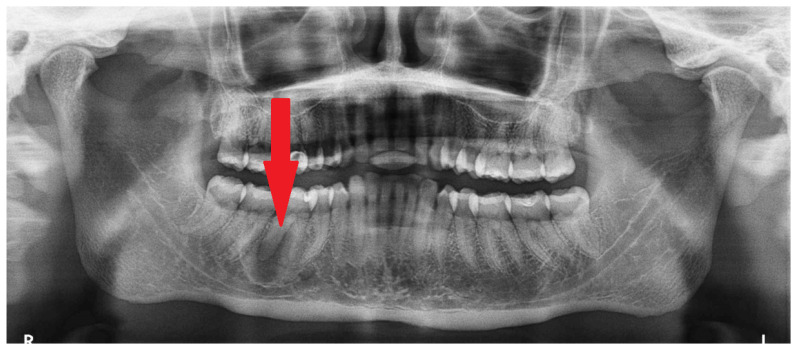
The occurrence of different irregular radiolucent areas on panoramic radiographs (panx) is quite a typical finding. Most are related to periapical teeth lesions, granulomas, odontogenic cysts, or other conditions. The occurrence of osseous, fibrous, or fibro-osseous lesions (cemento-osseous dysplasia, ossifying fibromas (their types psammomatoid, juvenile), and fibrous dysplasia (DF) in the mandible requires improved diagnostics with CBCT—cone beam computed tomography. A classic panoramic radiograph has many limitations in fully investigating each bone lesion in the jaw. Assessing the status of dental roots (displaced, resorption), the cortical buccal and lingual plates (expansion, swelling, erosion), the presence of periosteal reaction or elevation, and the shape, size, and status of adjacent teeth might be quite limited. The occurrence of ossifying fibroma (OSF) might be misdiagnosed as certain bone dysplasias, odontogenic cysts, or cemento-osseous dysplasia (COD) at various stages of maturation and calcification. It may also be mistaken for static bone cavities (SBCs) or even overlooked on panx. In some cases, similar bone cavities might be associated with other bone pathologies like brown tumors in hyperparathyroidism or other thyroid, parathyroid, and pituitary gland anomalies related to calcium–phosphorus metabolism [1,2,3]. The first step should always include improved diagnostics, followed by a scheduled biopsy—such as incisional biopsy—or lesion observation. Improved diagnostics may include SPECT (single-photon emission computed tomography) or, to exclude any metabolic disorders, a complete blood panel that examines calcium–phosphorus metabolites (serum calcium concentration (Ca), parathyroid hormone (PTH), active vitamin D (1,25(OH)2D), magnesium, blood phosphorus levels, bone alkaline phosphatase, thyroid hormones such as thyroxine (T4) and triiodothyronine (T3), and TSH [1,2,3]). The presented panx shows a radiolucent, irregular area under tooth 46 (the lower right first molar) without a clear border (red arrow), adjacent to the superior part of the mandibular canal, without any clear teeth resorptions or displacements.
